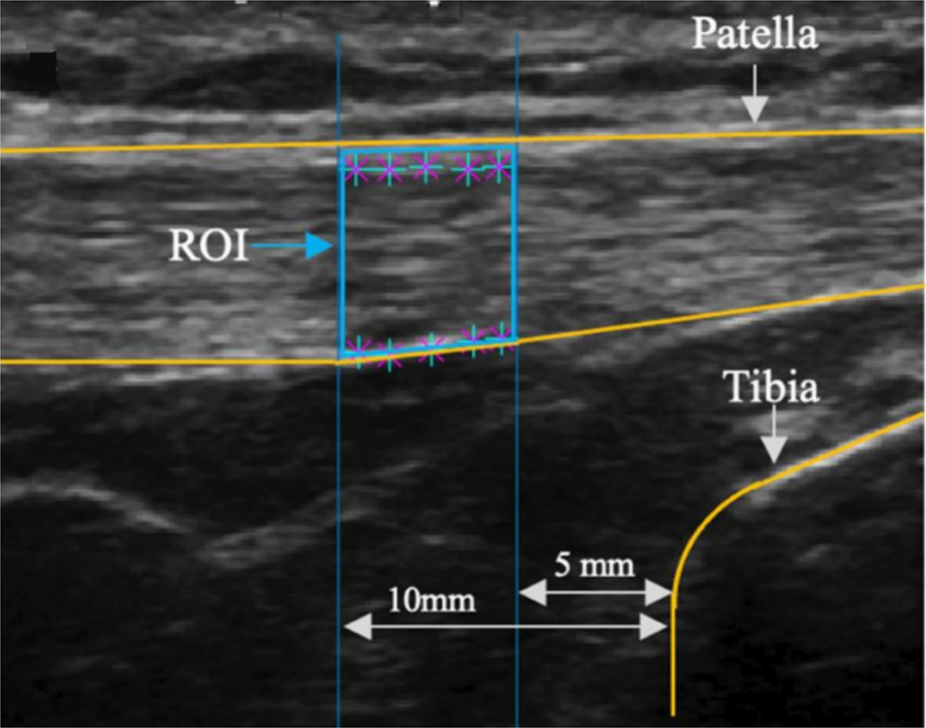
Ultrasound image of patellar tendon. ROI, region of interest.
